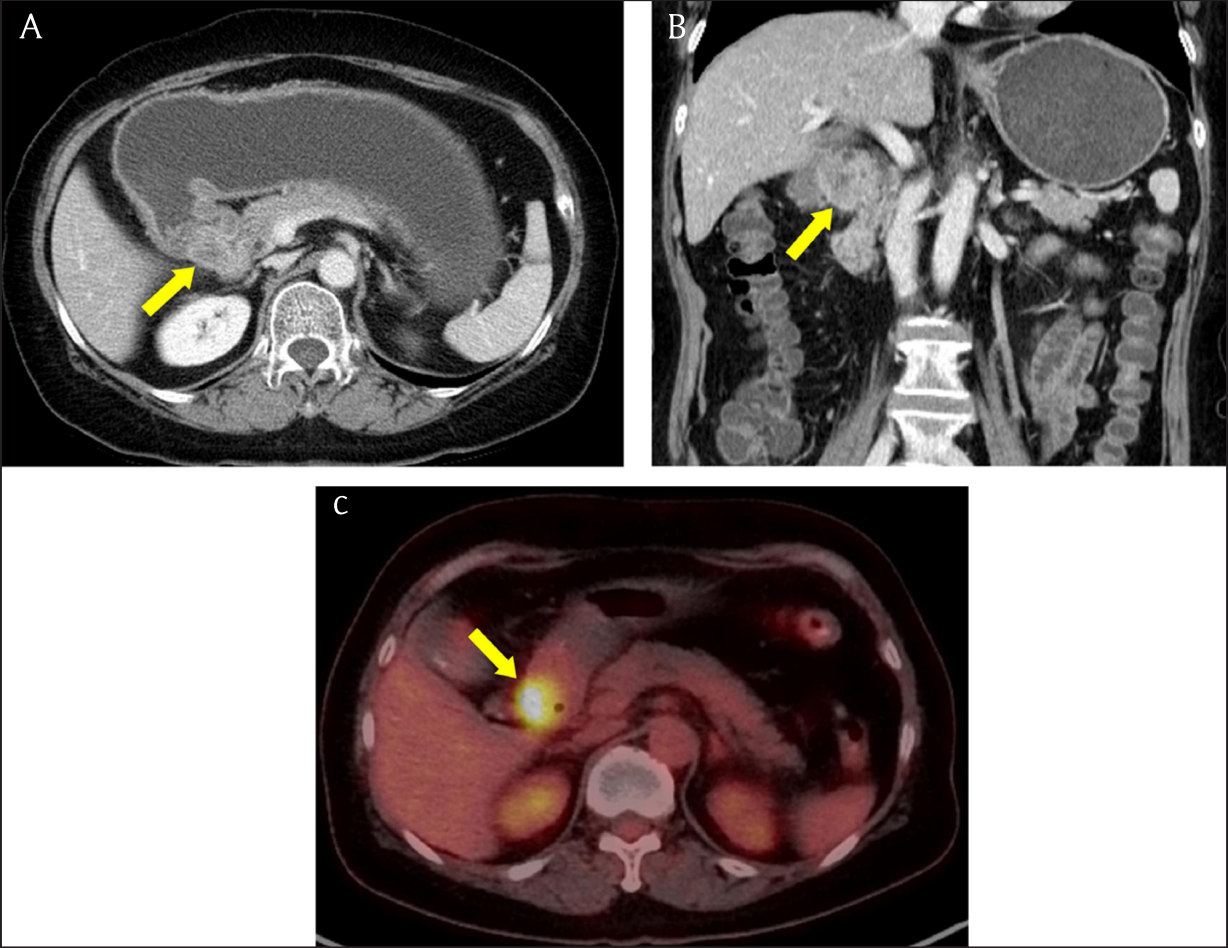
A 68-year-old woman with adenocarcinoma arising from heterotopic pancreas. (A, B) Axial and coronal reformatted CT images show irregular mass (arrow) with heterogeneous enhancement in gastric pylorus. (C) Positron emission tomography-CT image shows intense uptake in the mass (arrow).
